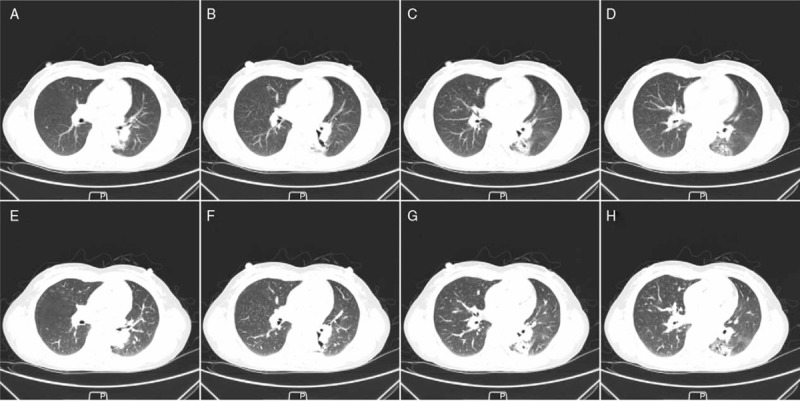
Chest CT images. The area assumed to be bronchiectasis in the lower left lung showed little change over >2 years. A–D, Chest CT images taken in 2014; E–H, chest CT images taken in 2016. CT = computed tomography.
